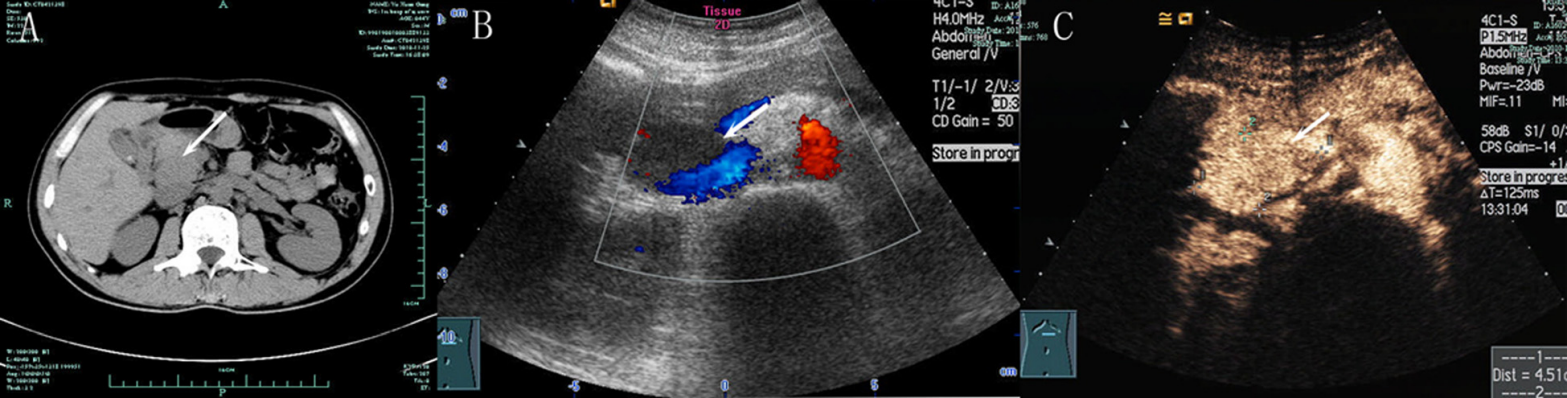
Image findings of case 1. Case1: Axial unenhanced computed tomography of abdomen depicts a 3 × 2.1cm mass (arrows) at pancreatic head (A). Contrast-enhanced ultrasonography reveals a solitary mass that homogeneous intense enhancement after contrast material administration (B,C).
